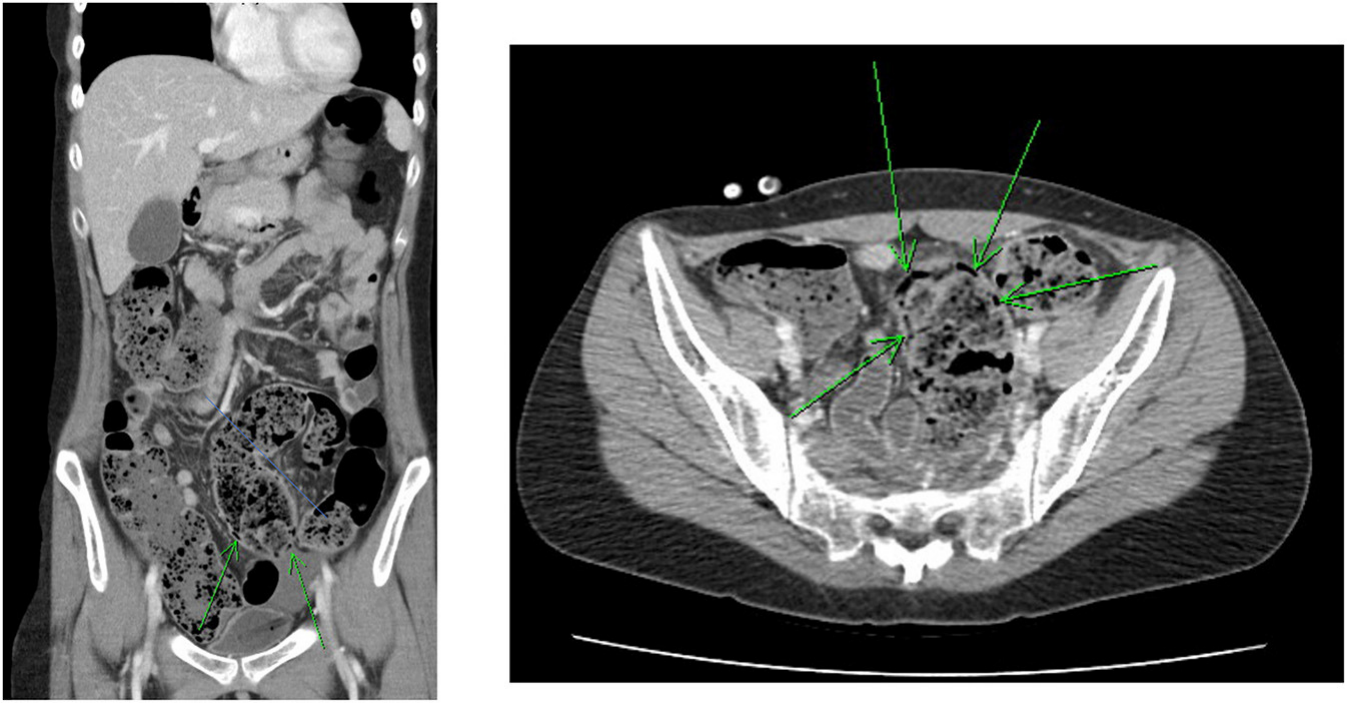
Abdominal CT revealed much fecal impaction and colon dilatation along with colon wall thickening and pneumatosis intestinalis (arrow).
